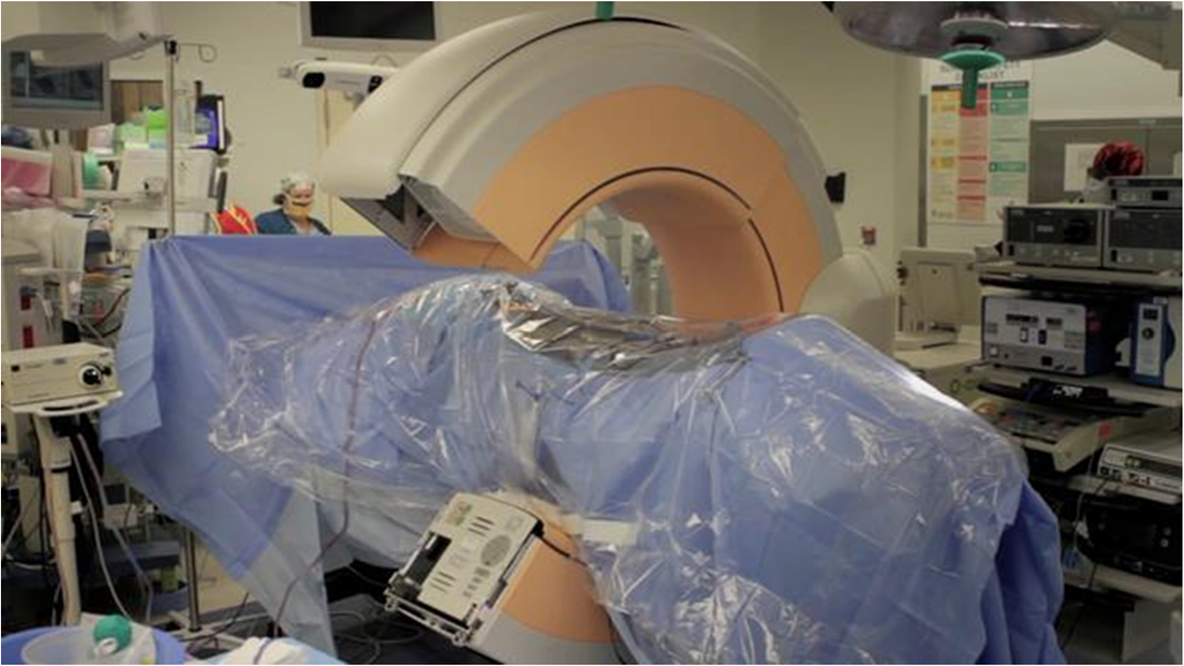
Typical intraoperative CT setup
